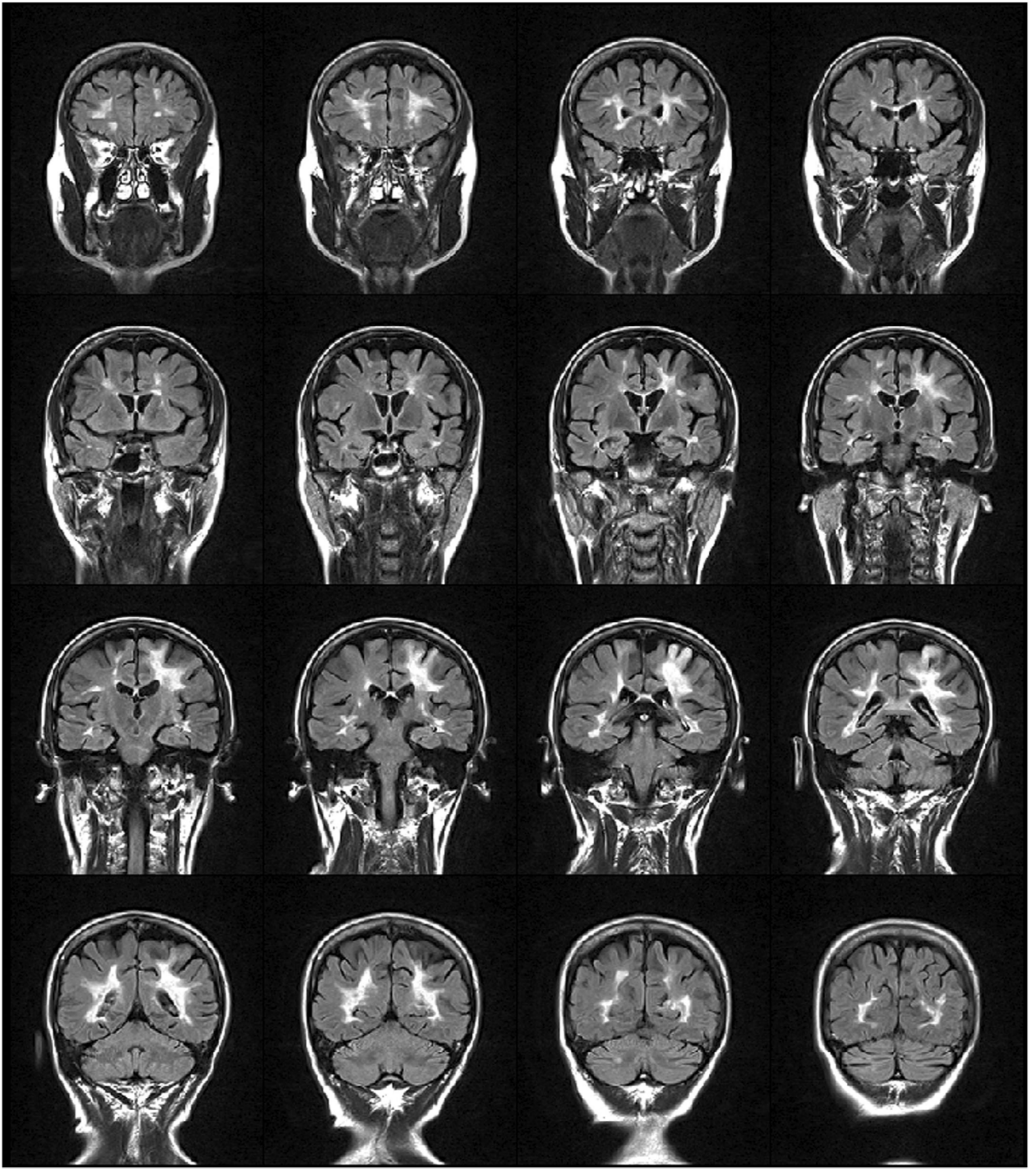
FLAIR weighted image showing multiple sclerosis lesions distributed mainly periventricularly and a hyperintense lesion in the fronto-parietal region that extends to the cortex, suggestive of progressive multifocal leukoencephalopathy.
